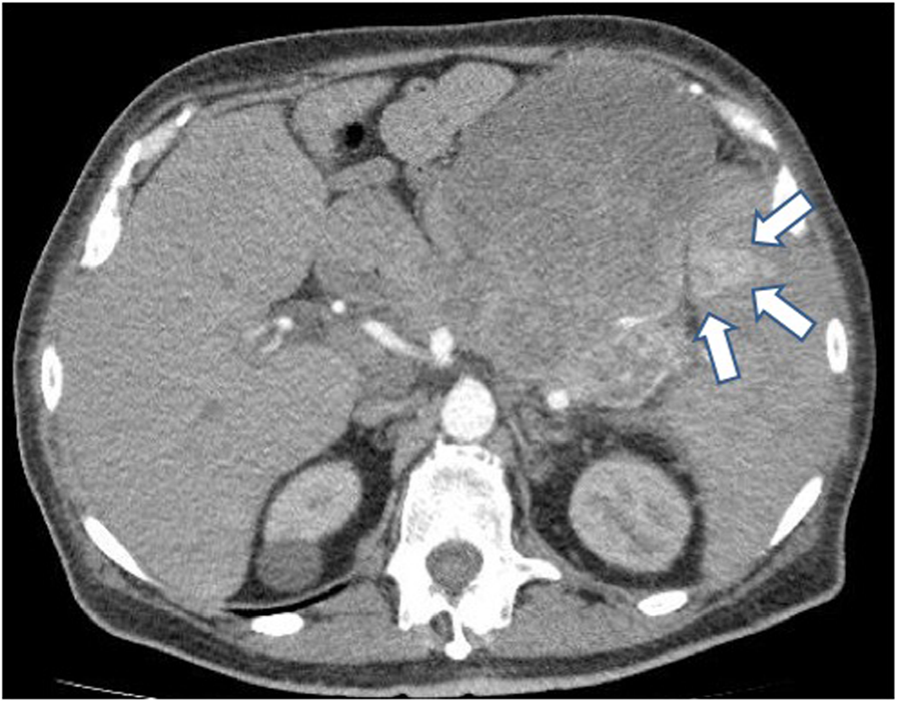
Preoperative computed tomography scan of the abdomen shows a 24 cm mass in the left upper quadrant between the spleen and liver, and a single metastatic lesion (1 cm) in the spleen (*arrows*)
